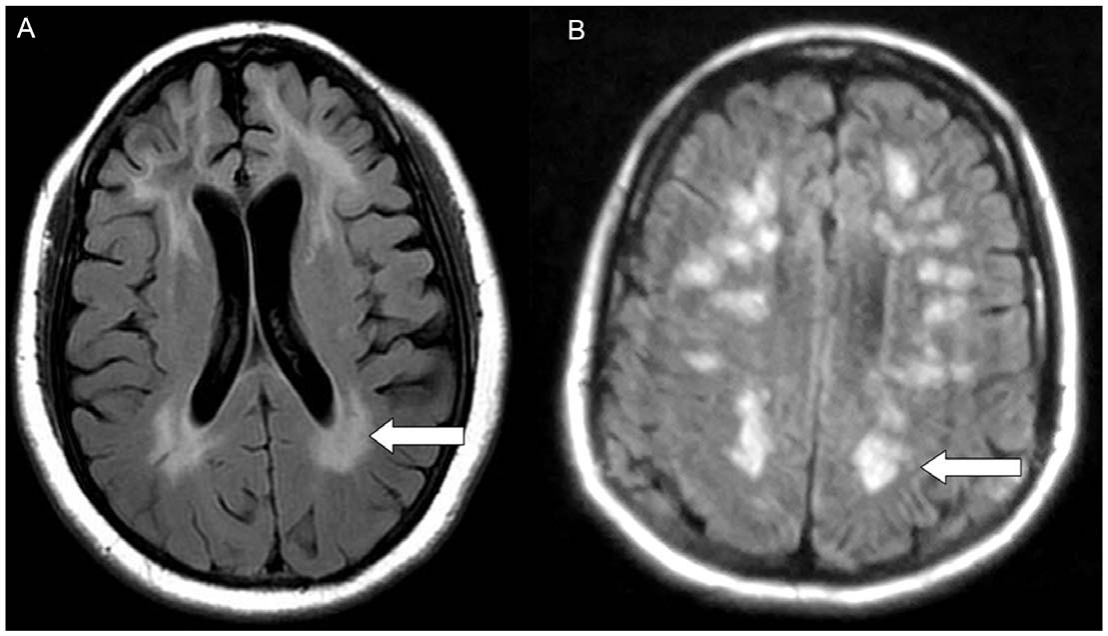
Leukoencephalopathy-like manifestations on MRI images. (A, B)Axial MRI images demonstrate widespread periventricular hyperintense signals in the cerebral white matter.
